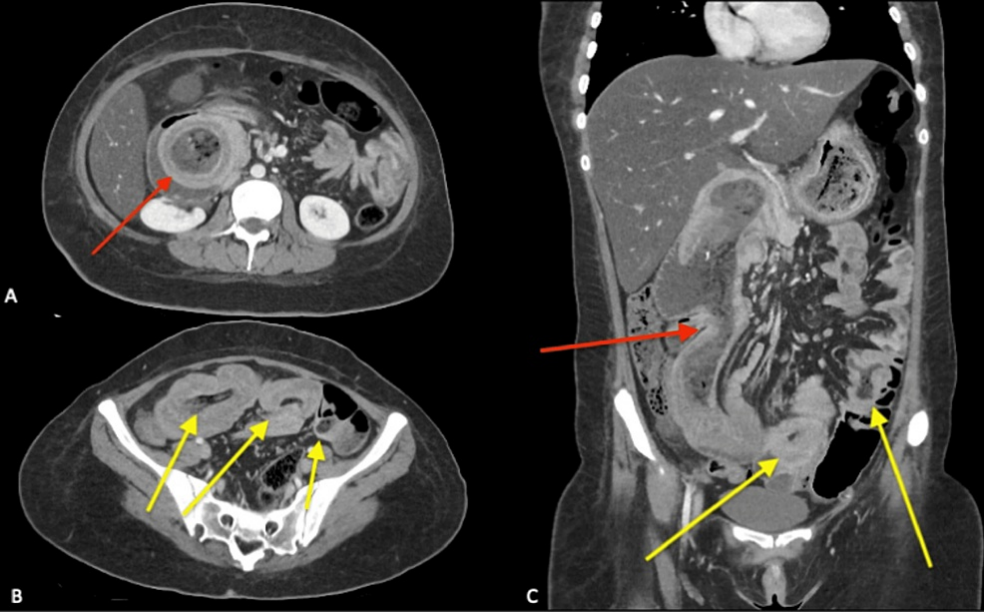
CT scan demonstrating multiple intussuscepting points. Red: Gastroduodenal intussusception. Yellow: Multiple separate jejuno-jejunal intussusception points
